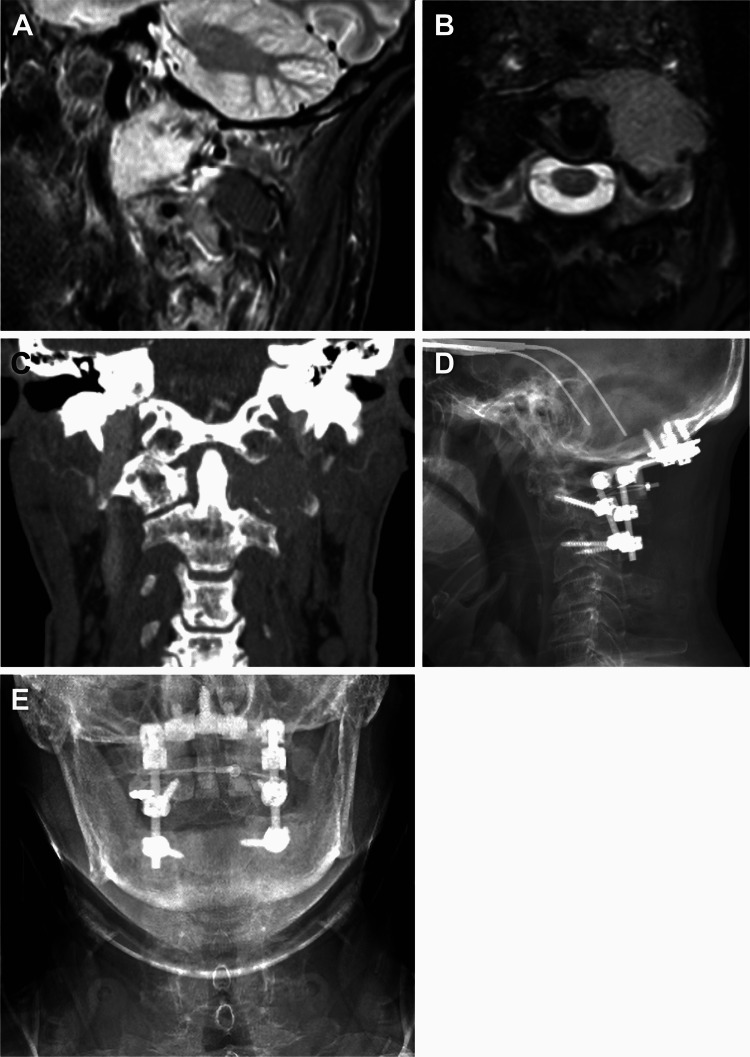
A man in his mid-50s presented with a bony lesion eroding the left C1 lateral mass. Preoperative sagittal (A) and axial (B) MRI showed a lesion in the left C1 lateral mass. (C) Preoperative coronal CT showed the lytic bone lesion. Biopsy results confirmed the diagnosis of plasma cell myeloma. The patient underwent fixation from the occiput to C3 with pedicle screws placed at the distal fixation point at C3 using the freehand technique with direct visualization. Three-month postoperative lateral (D) and anterior (E) radiographs showed intact hardware. Used with permission from Barrow Neurological Institute, Phoenix, Arizona.
